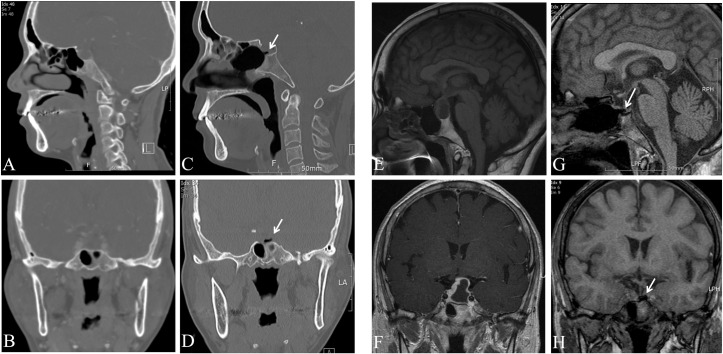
Pre- and post-operartive images of patient showing invagination of the sphenoid sinus mucosa. (A,B) Pre-operartive PNS CT; sagittal and coronal setting. (C,D) Post-operartive PNS CT; sagittal and coronal setting. (E,F) Pre-operartive PNS MRI T1; sagittal and coronal setting. (G,H) Post-operartive PNS T1; sagittal and coronal setting. Arrows indicate invaginated lesion of the sphenoid sinus mucosa.
